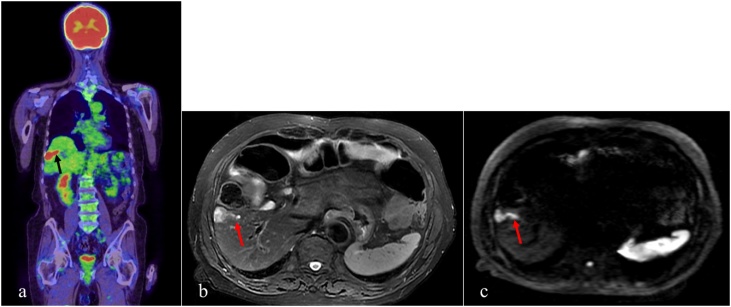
Preoperative images. a) Positron emission tomography image. b) Magnetic resonance (MR) T2-weighted image. c) MR imaging diffusion-weighted image. Glisson’s pedicle infiltration (arrow) was suspected on the basis of these images.
